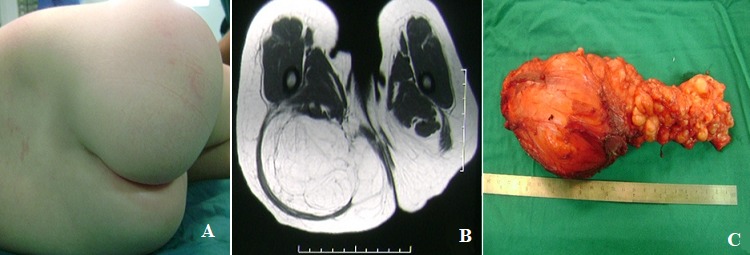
A) tumefaction of the right buttock, it was soft consistency and mobile in relation to the deep planes; B) pelvic MRI showed a large fatty lobulated mass well encapsulated with septa, with low signal T1/T2; C) pathological examination of the resection piece confirmed the diagnosis of lipoblastoma
